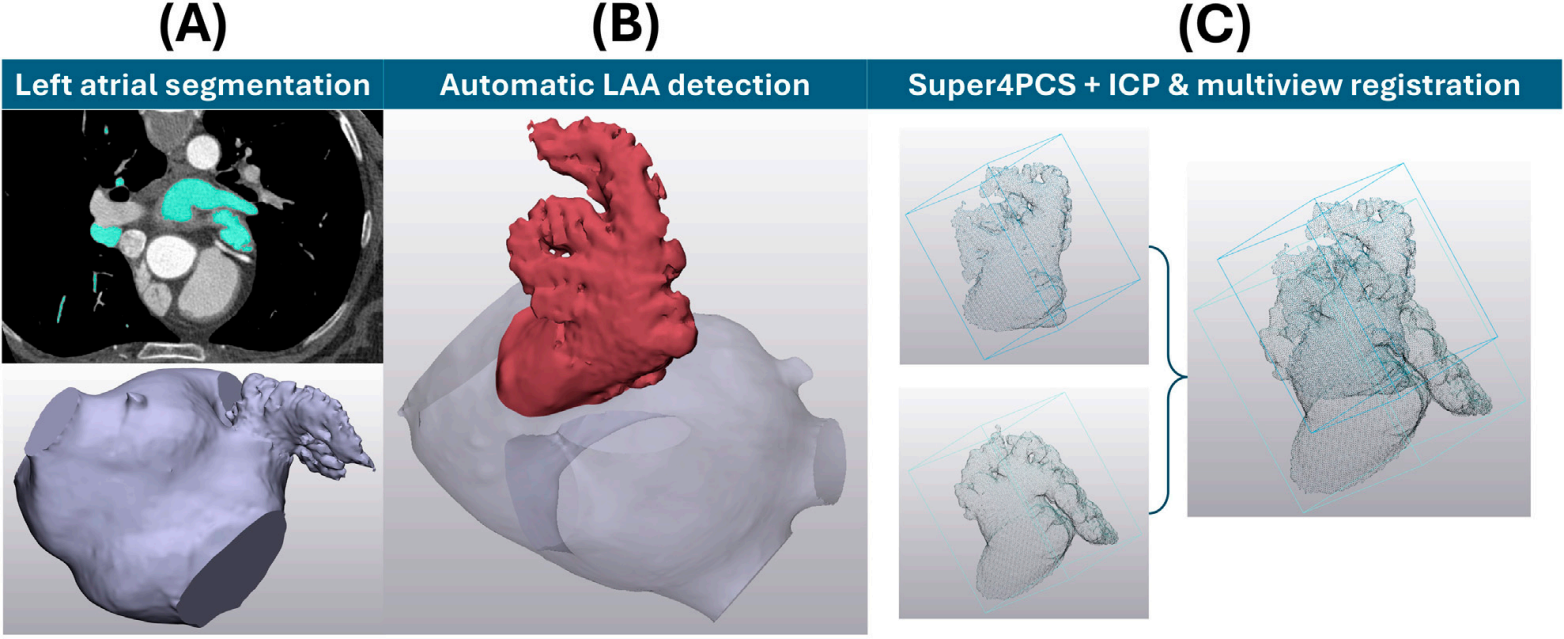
(A) LAA mesh acquisition and processing prior to SSA and clustering. The upper far left shows an example slice of the CT image stack to achieve the lower left atrial segmentation. (B) The LAA position determined through a fully automatic detection algorithm (Martorana et al.). (C) Two examples of LAA point clouds before and after alignment through Super4PCS registration, followed by ICP & multiview registration of all possible pairs.
